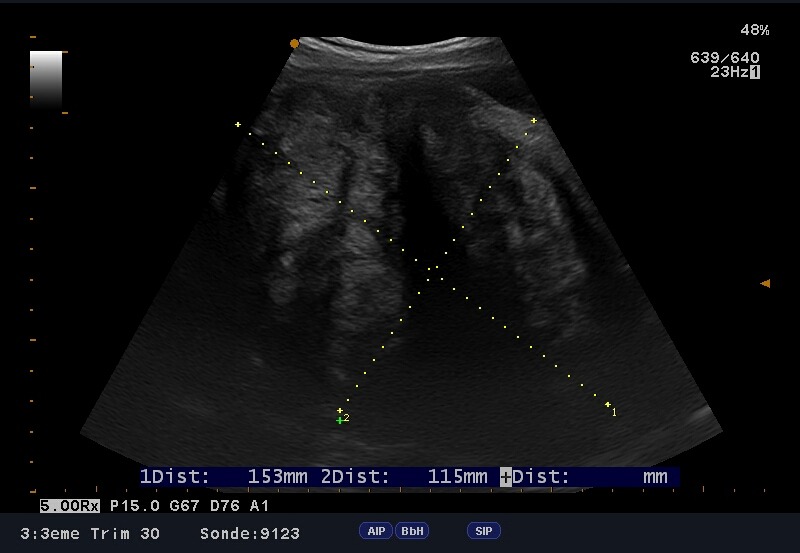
Sonographic image of fetal measurement
